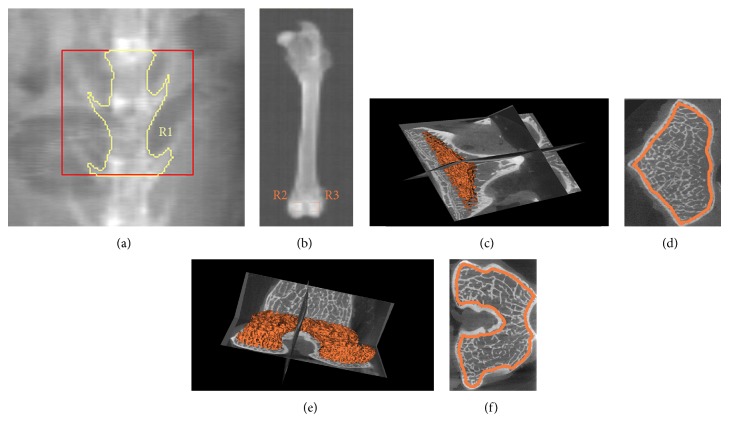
DXA and *μ*CT measurement of vertebrae and femoral condyles. (a) DXA measurement of vertebrae. R1: ROI of L3 and L4. (b) DXA measurement of femoral condyles. R2 and R3: ROI of femoral condyles (7 mm × 7 mm ROI). (c) The 3D reconstruction of the ROI in vertebrae in *μ*CT measurement. (d) The ROI on the axial section of vertebrae in *μ*CT measurement. (e) The 3D reconstruction of the ROI in femoral condyles in *μ*CT measurement. (f) The ROI on the axial section of femoral condyles in *μ*CT measurement. DXA: dual-energy X-ray absorptiometry; ROI: regions of interest.
